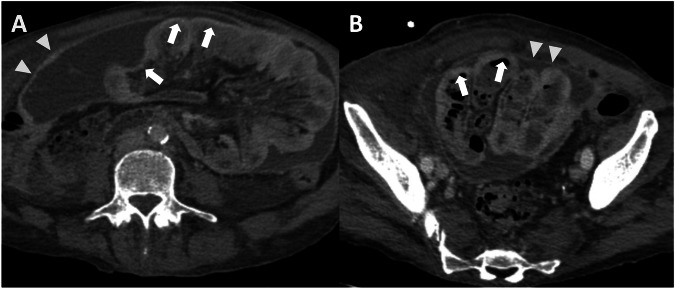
Malignant pleural mesothelioma with peritoneal implants in a 67-year-old man. CT delayed phase and portal-venous phase (A, B), axial plane. Small intestine loops were completely covered by a thickened layer of visceral peritoneum (arrowheads) known as the “Teca aspect.” Moreover, the parietal peritoneum is thickened (arrows)
